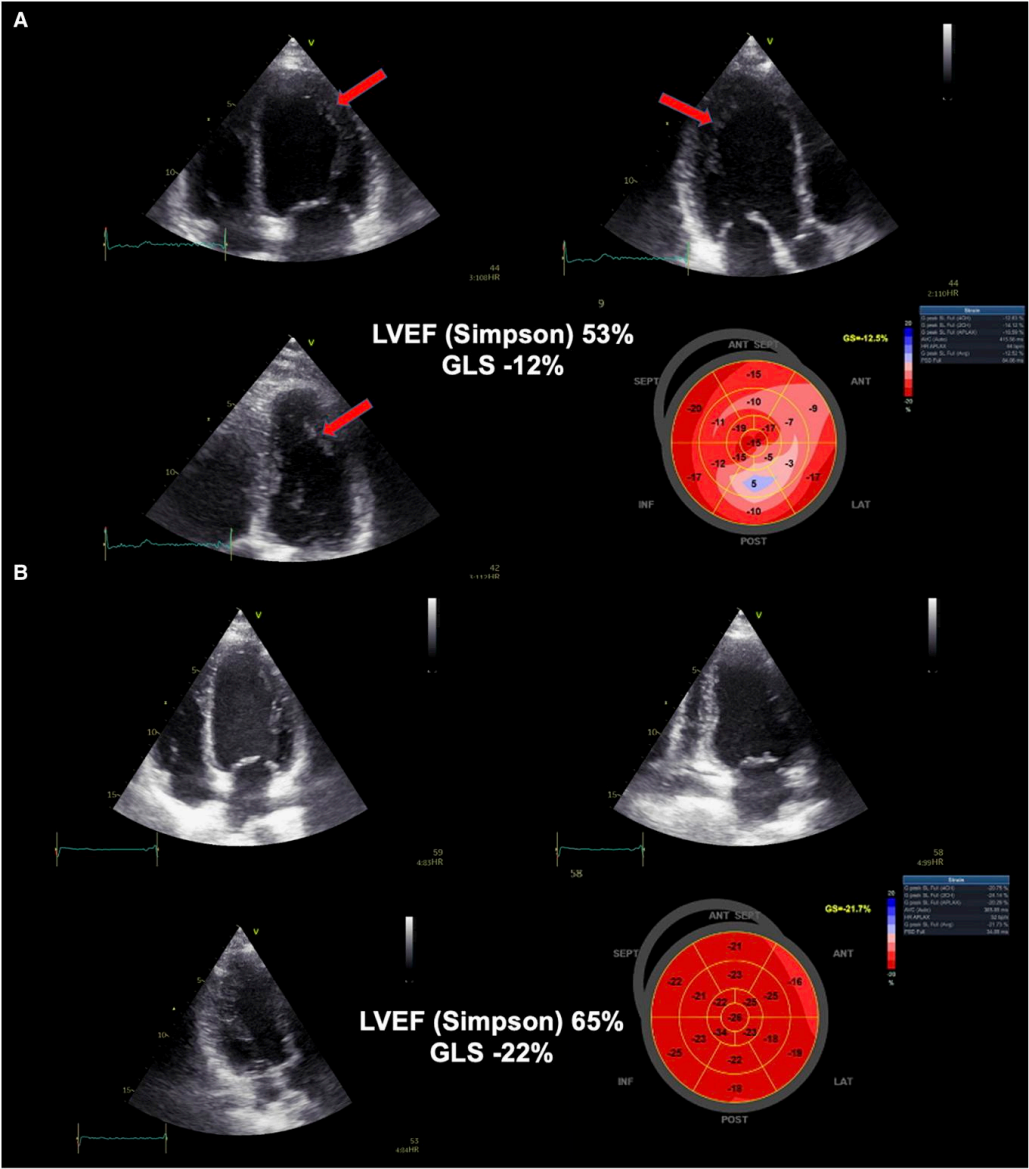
A transthoracic echocardiography examination at (*A*) index hospitalization and (*B*) follow-up examination at 1 month following discharge. The image depicts 2-chamber and 4-chamber views of the heart with ‘bullseye’ plot showing global longitudinal strain values. Red arrows show trabeculation within LV structure.
